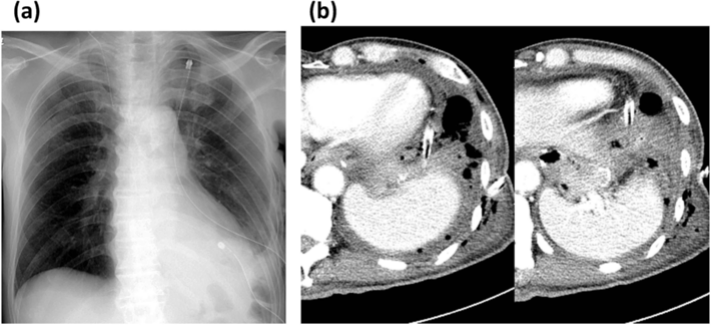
a Chest X-ray showed good expansion of the remaining left lung. b Chest computed tomography showed fluid collection around the chest drainage tube right above the diaphragm
